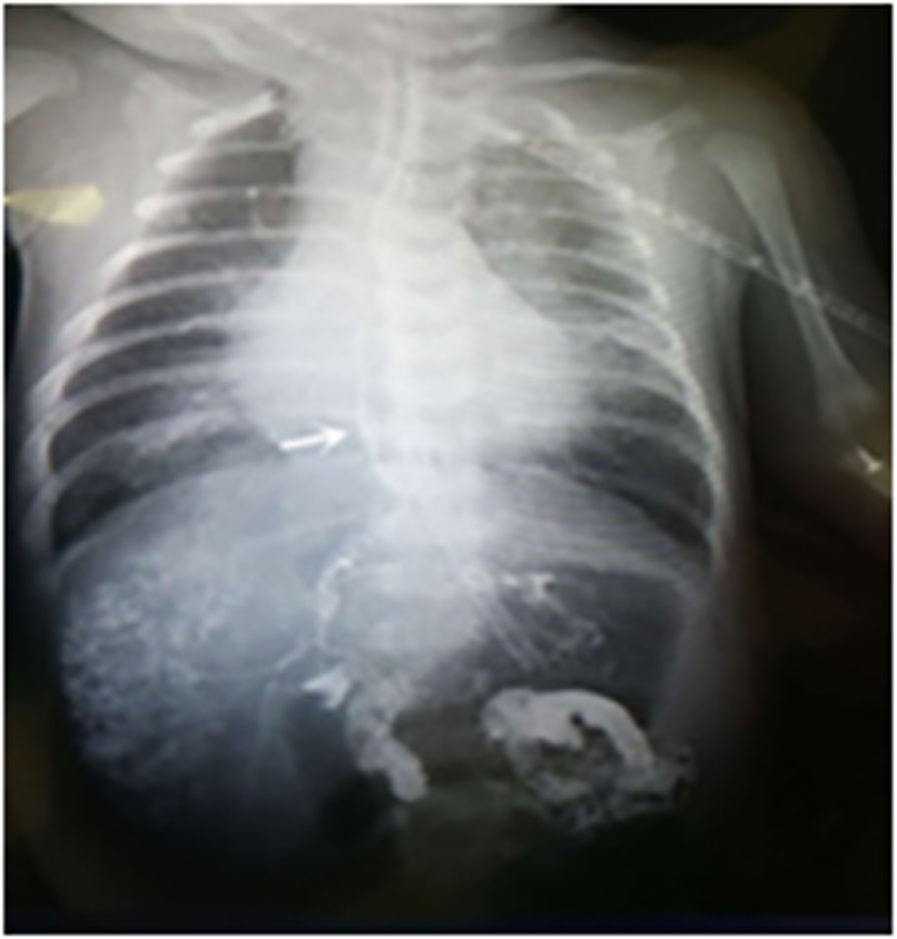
Bedside x-ray showing contrast flowing from the abnormal opening in the right main bronchus through the fistula and into the biliary tract, the gall bladder, and the duodenum. Contrast study via fiberoptic bronchoscope: contrast is seen in the fistula, the biliary tract, and the duodenum. The white arrow shows the fistula
